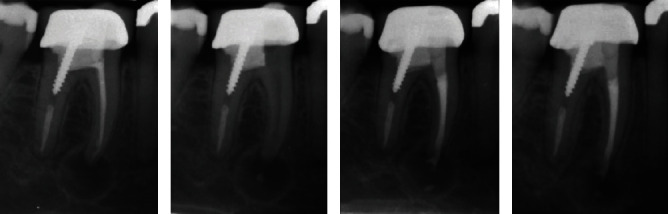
A mandibular right first molar with symptomatic apical periodontitis. (a) Diagnostic periapical radiography showing compromised previous endodontic treatment and a large periapical radiolucency around the mesial root. (b) In-treatment periapical radiography demonstrating conservative preparation of access cavity, removal of obturation materials, and proper cleaning and shaping of the mesial canals. (c) Immediate post-treatment radiography, revealing periapical lesion regression with clinical disappearance of pain and relative healing of the regional swelling. (d) Post-operative radiograph after 10 months of the initial treatment, exhibiting bone healing and progressive disappearance of the large periapical radiolucency.
